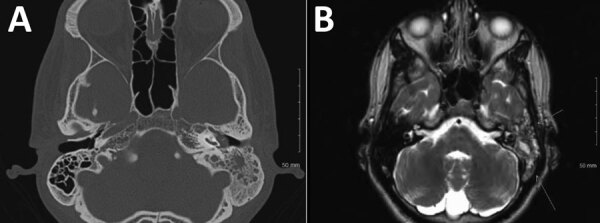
Radiographic findings from 22-year-old Hispanic man from California, USA (case 4), in multicentric case series of coccidioidal otomastoiditis. A) Computed tomography scan of the head, showing opacification of the mastoid. B) Magnetic resonance image of brain, showing mastoiditis.
